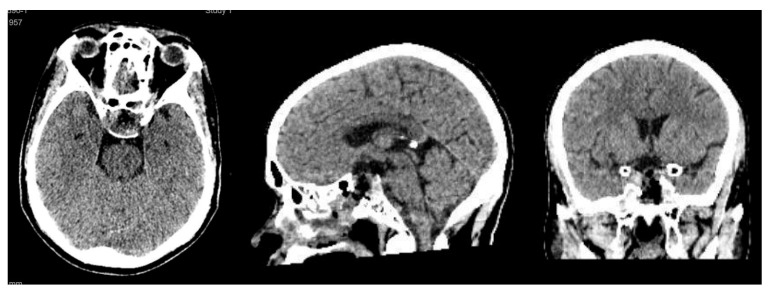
Axial, sagittal, and coronal CT scan performed on postoperative Day 1 after surgery.
